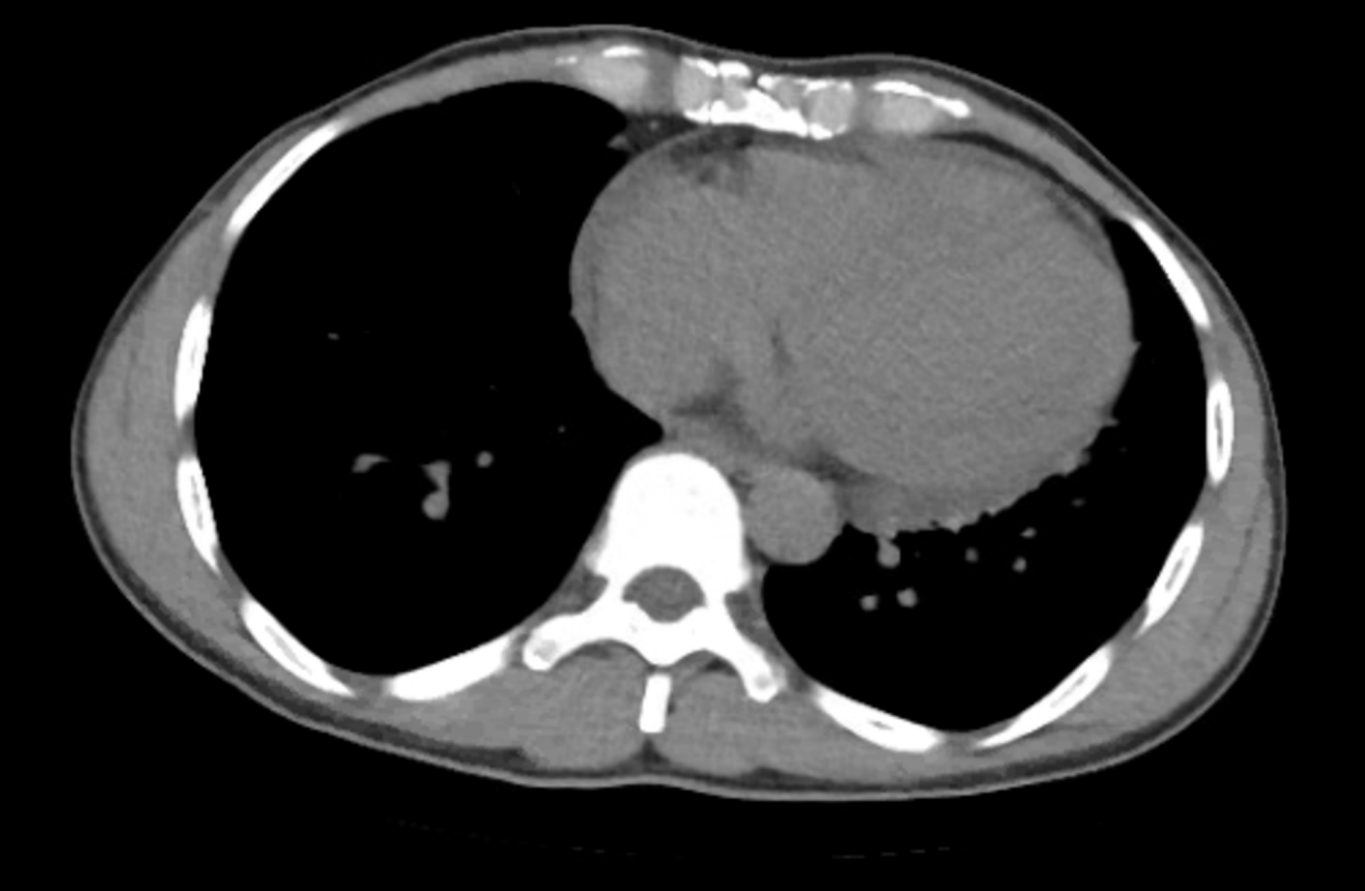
Admission computed tomography (CT) scan showing cardiomegaly
